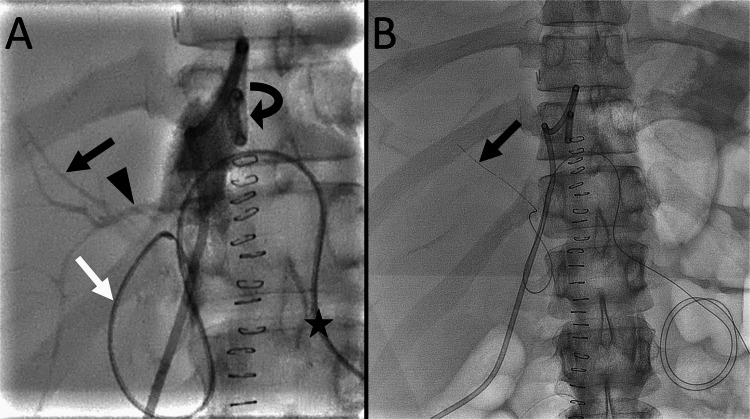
Percutaneous biliary drainage tube placement in the right anterior segmental bile duct (A) A frontal radiograph with a contrast injected through the drainage tube (curved arrow) in the biloma shows the segment 8 bile duct (black arrow) and the right posterior hepatic duct (arrowhead). A seeking catheter (white arrow) is advanced from the blind end of the jejunum. The catheter is inserted through the blind end of the jejunum (star). (B) A frontal radiograph shows a drainage tube (arrow) placed in the segment 8 bile duct.
